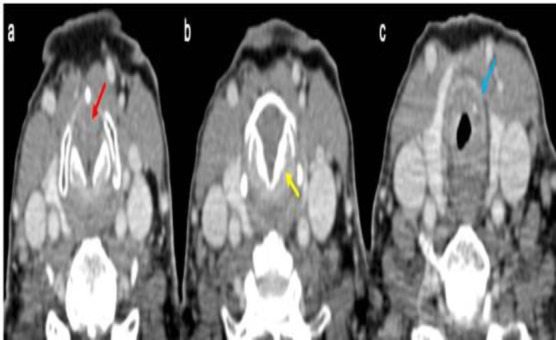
CT images of glottic-subglottic stenosis: (a) glottic plane (red arrow), (b) subglottic plane showing cricoid cartilage thickening (yellow arrow), (c) first tracheal ring (blue arrow).
